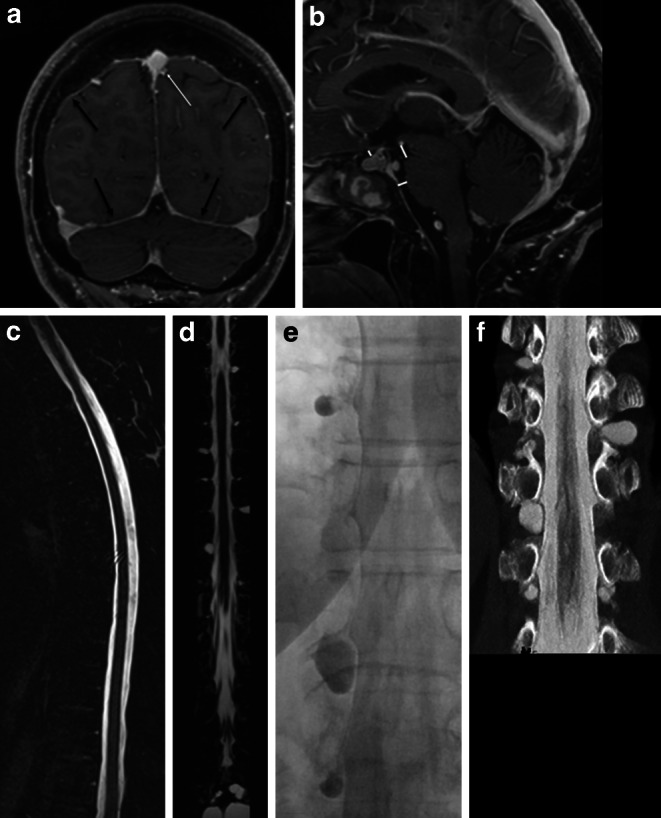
Patient with orthostatic headache. a, b Brain MRI demonstrating pachymeningeal enhancement (a; *black arrows*), venous engorgement (a; *white arrow*), no subdural collection, effaced suprasellar (< 4.0 mm) and prepontine (< 5.0 mm) cistern, and decreased mamillopontine distance (< 6.5 mm); SIH score = 8 indicating high likelihood of SIH. c, d Spine MRI does not show a spinal longitudinal extradural CSF collection; however, multiple spinal nerve root cysts are demonstrated. e, f Conventional dynamic myelography and postmyelography CT demonstrate filling of the nerve root cysts without epidural contrast agent leakage or a CSF venous fistula. *CSF* cerebrospinal fluid, *CT* computed tomography, *MRI* magnetic resonance imaging, *SIH* spontaneous intracranial hypotension
